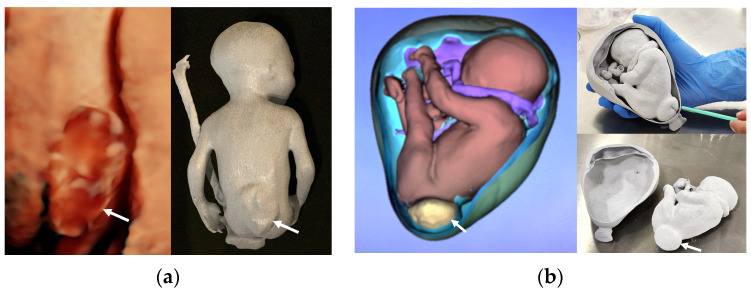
(a) Fetus with myelomeningocle and Chiari II malformation at 23 weeks’ gestation. Three-dimensional ultrasound and printed model built in a powder-based system showing myelomeningocele (arrow); (b) virtual and printed model (25 weeks) in powder system for medical training.
